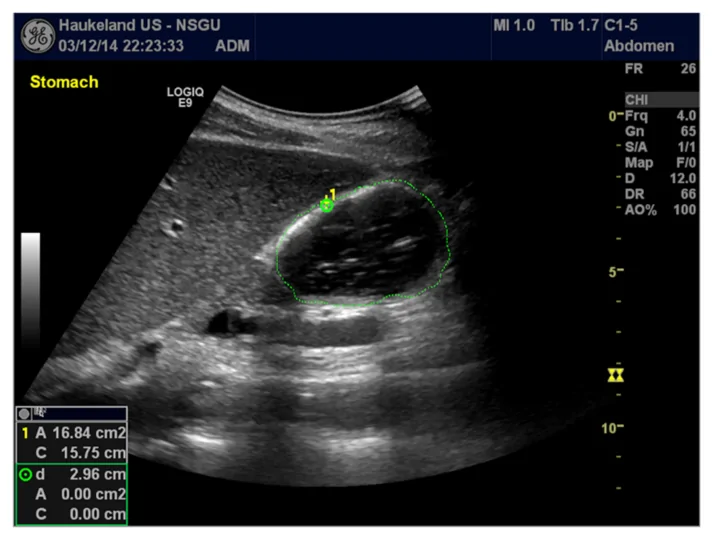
A 2D ultrasonographic image showing the gastric antrum area. The antrum is measured in the sagittal plane, using the aorta and superior mesenteric vein as anatomical landmarks. An increased gastric antrum area may be associated with dyspepsia.
